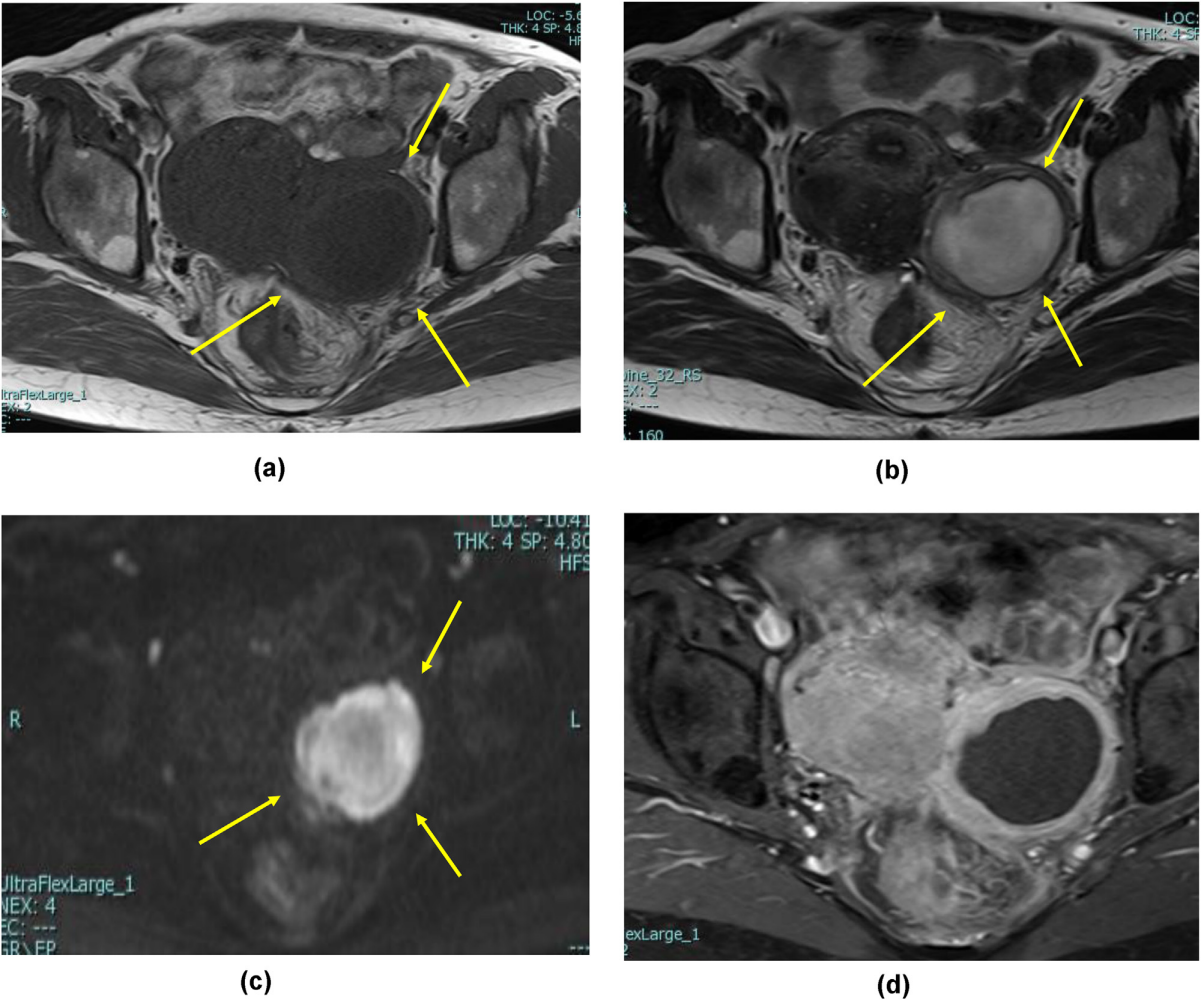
MRI findings of ovarian abscess. Yellow arrow indicates the location of the left ovarian tumor (A) T1-weighted image showing a pale wall and high signal intensity (B) T2-weighted image showing high signal intensity (C) Diffusion-weighted image showing high signal intensity and (D) The abscess wall appears enhanced by the gadolinium contrast.
